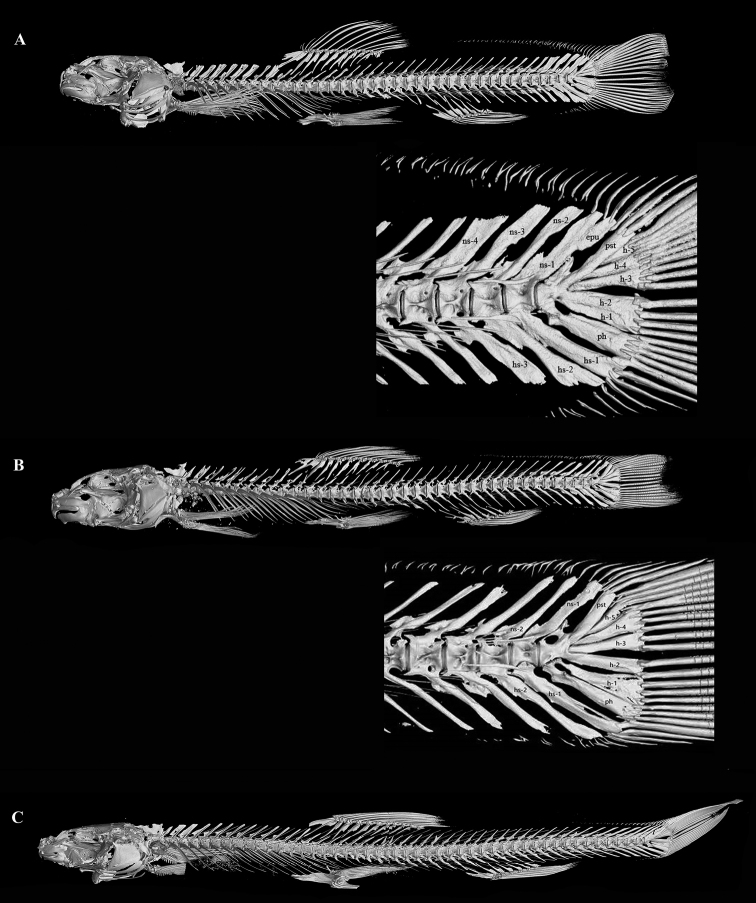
Lateral CT scans of: A*H.robusta* sp. nov., KIZ 2009000125, lateral view and structure of caudal skeletons B*H.nanpanjiangensis*, KIZ 1994000029, lateral view and structure of caudal skeletons C*H.wenshanensis*, KIZ 2014005686, lateral view. Abbreviations: ph—partypurale, ns—neural spine, hs—haemal spine, h—hypural, epu—epurale, pst—pleurostyle.
